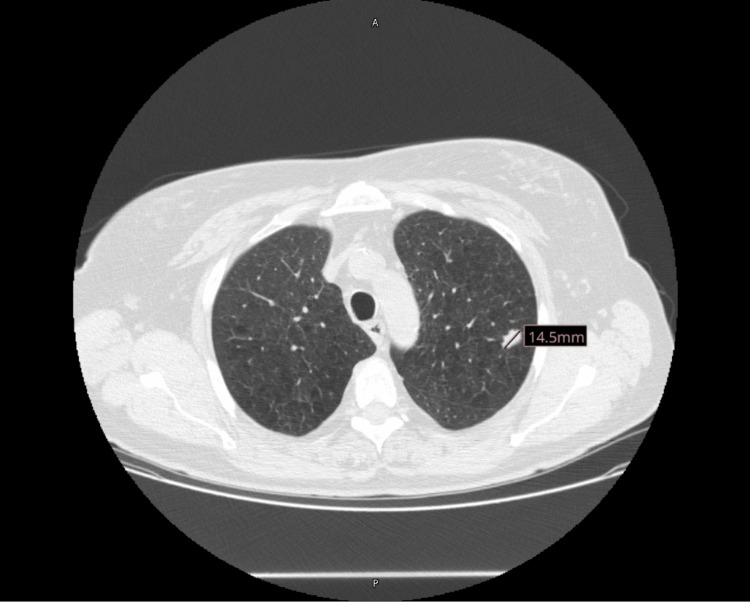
The initial chest CT scan shows a 1.4 cm spiculated left upper lobe nodule.
